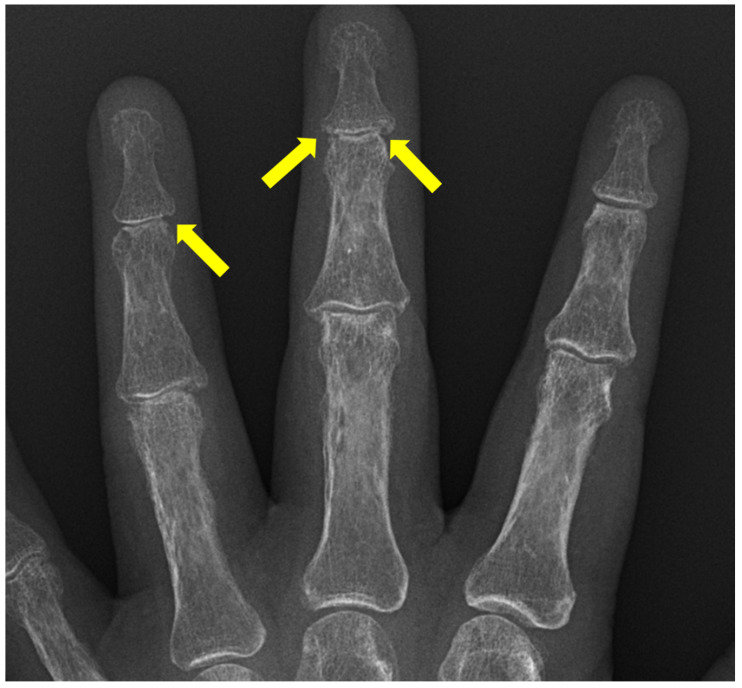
59-year-old female with psoriatic arthritis. Hand radiographs show marginal erosions in the distal interphalangeal joints of the index and middle fingers (yellow arrows). Mild periosteal reaction is seen at the middle finger DIP joints.
